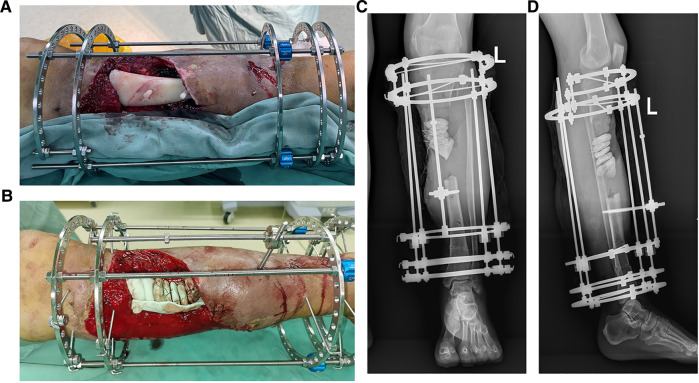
The intraoperative application of PSI and postoperative x-ray images in ilizarov technique of distraction osteogenesis in case 1. (A) The PSI was put into the bone defect to maintain the alignment of the fracture fragments. (B) Several pie-shaped bone cements were stacked into the defect which would be gradually withdrawn according to the bone transportation. (C) Postoperative x-ray on anterior-posterior (AP) view of case 1, the angulation of valgus is 1.74°. (D) Postoperative x-ray on lateral view after operation of case 1, the angulation of anteversion is 1.14°.
